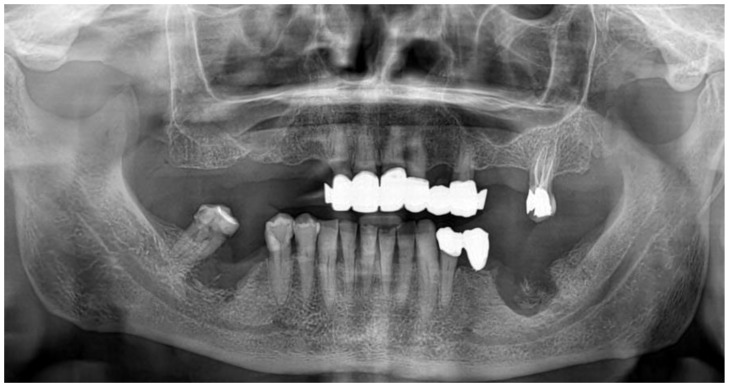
The panoramic radiograph showing the two regions affected by MRONJ.
